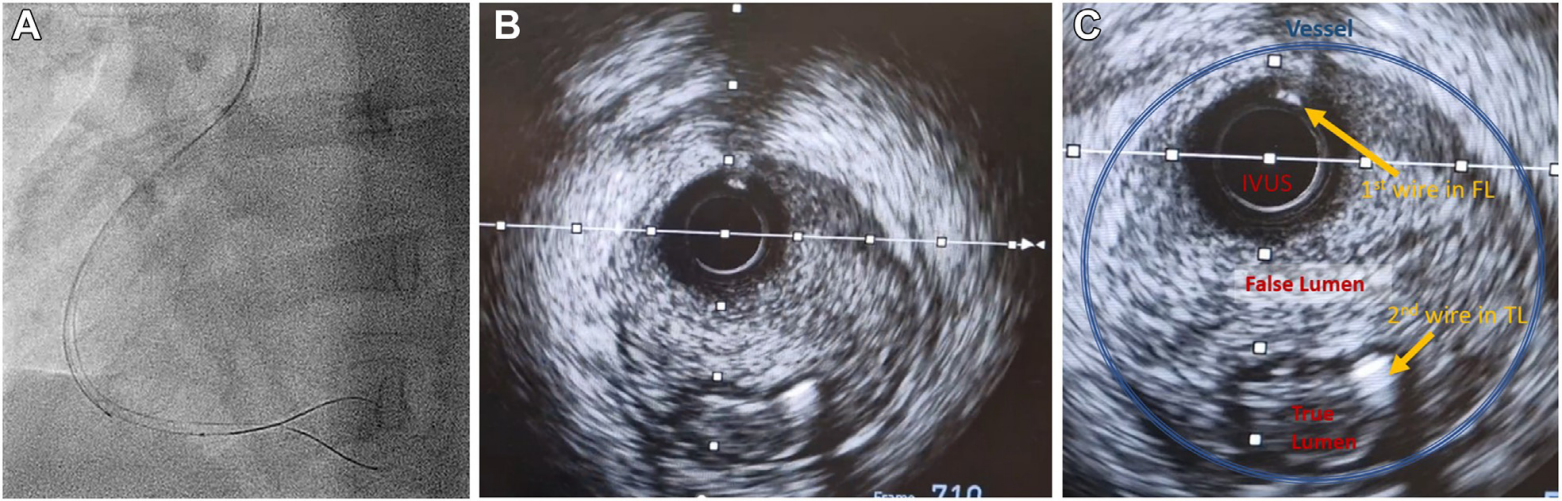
Angiography and Intravascular Ultrasound (A) Second guidewire on angiography in the true lumen (TL) on (B and C) intravascular ultrasound with the original wire in the false lumen (FL).
